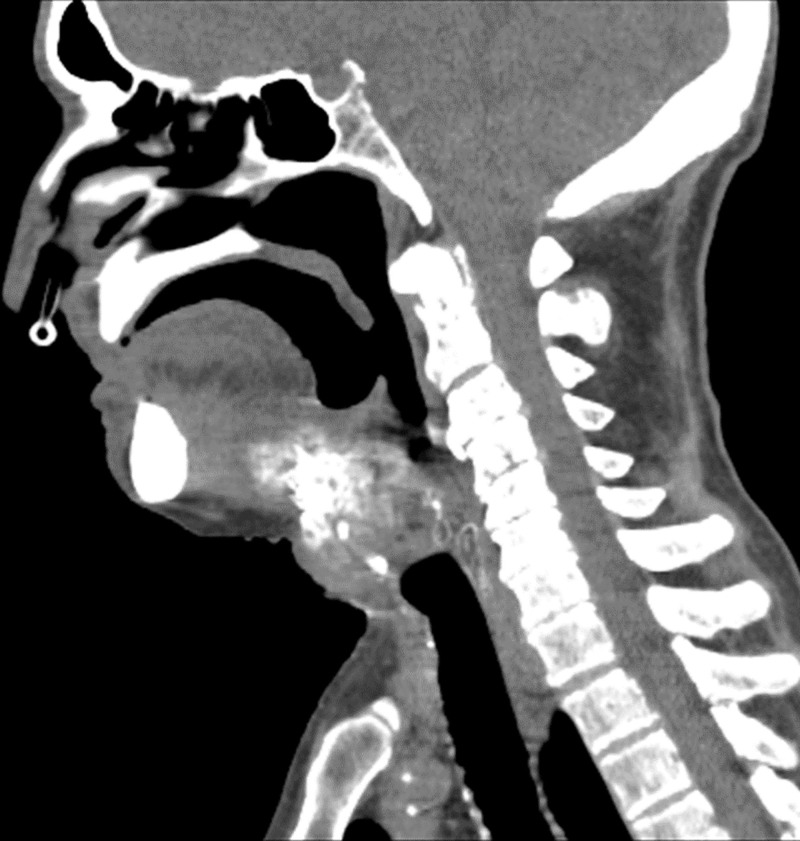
Sagittal CT demonstrating a calcified subcutaneous mass at the hyoid level, extending to the thyroid cartilage and tongue base.
